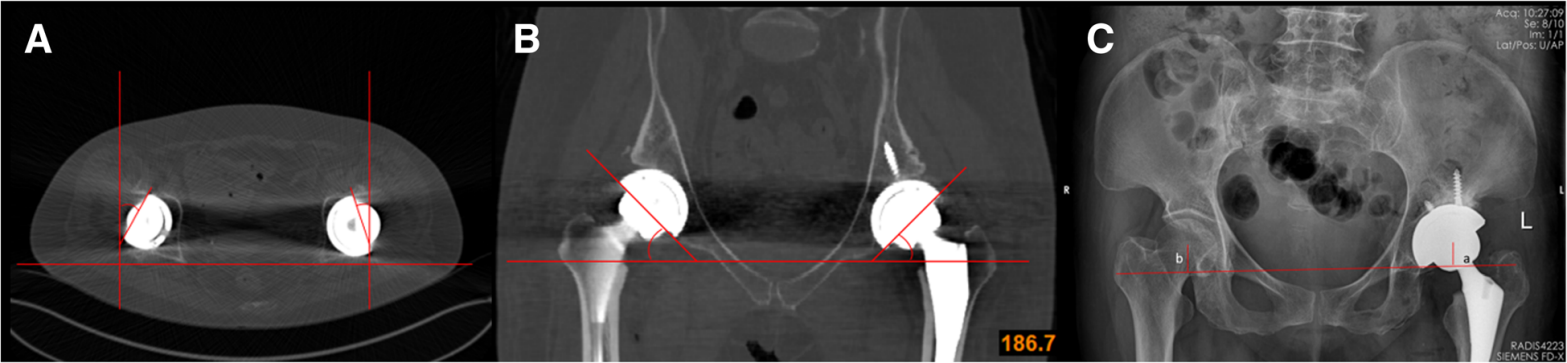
We used computed tomography to present the transverse view to measure the anteversion (a) and the coronal view (b) to measure the inclination. We used a standard pelvis anterior-posterior X-ray (c) to assess the BRCD (BRCD = |b-a|). a and b represent the distance between the rotator center and the line that passes through the bilateral teardrop
